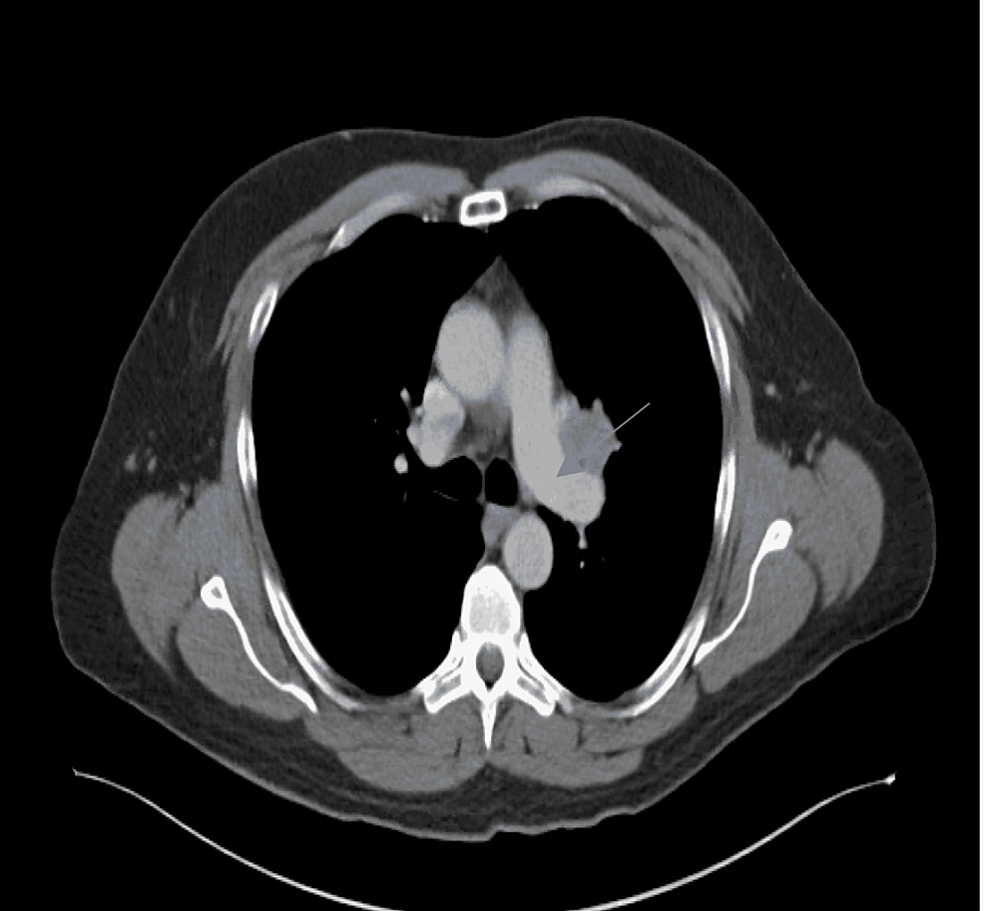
Computed Tomography (CT) of the chest demonstrating a 2.2 cm x 2.8 cm left perihilar stellate-shaped mass lesions with a probable invasion of adjacent lingular bronchus and possibly left main pulmonary artery.
